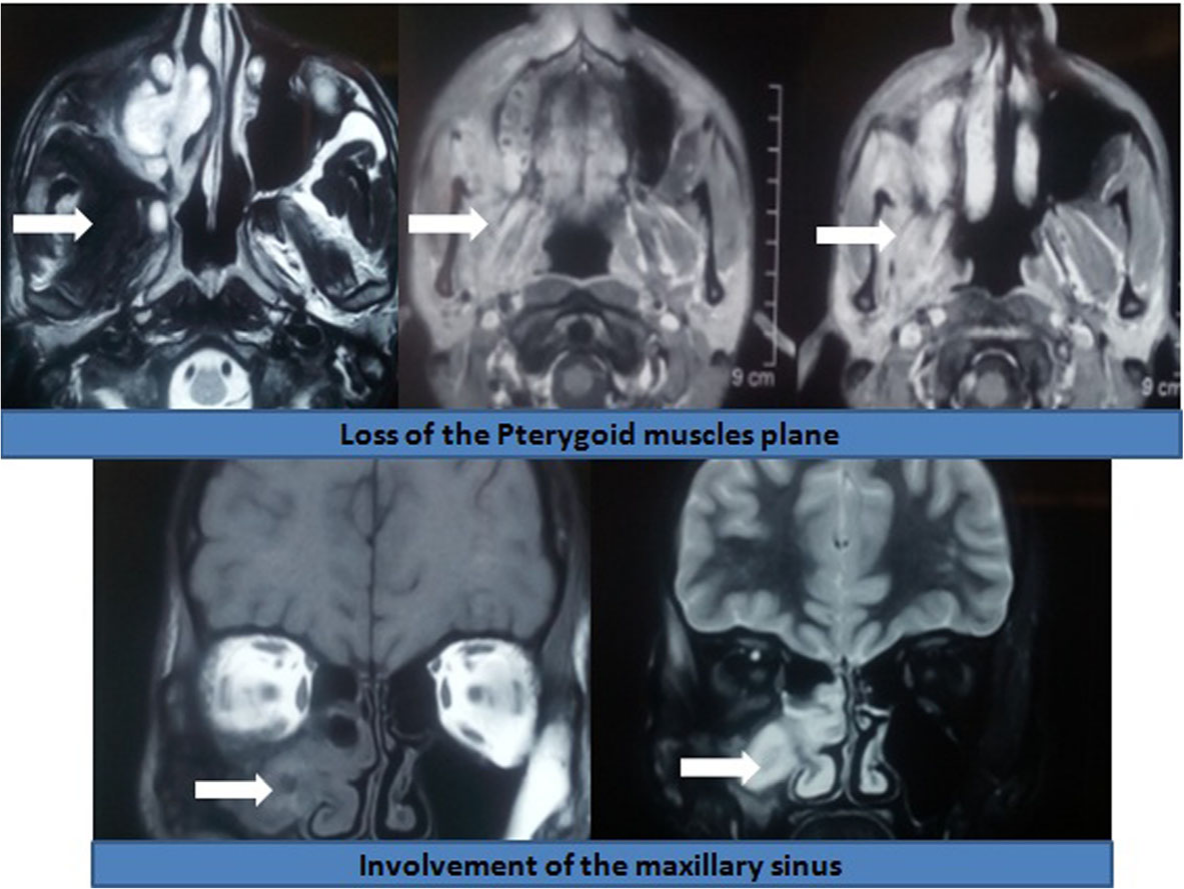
Magnetic resonance images showing the extension of the lesion with characteristic findings in different image sequences (as depicted by arrowheads in respective image panels)
